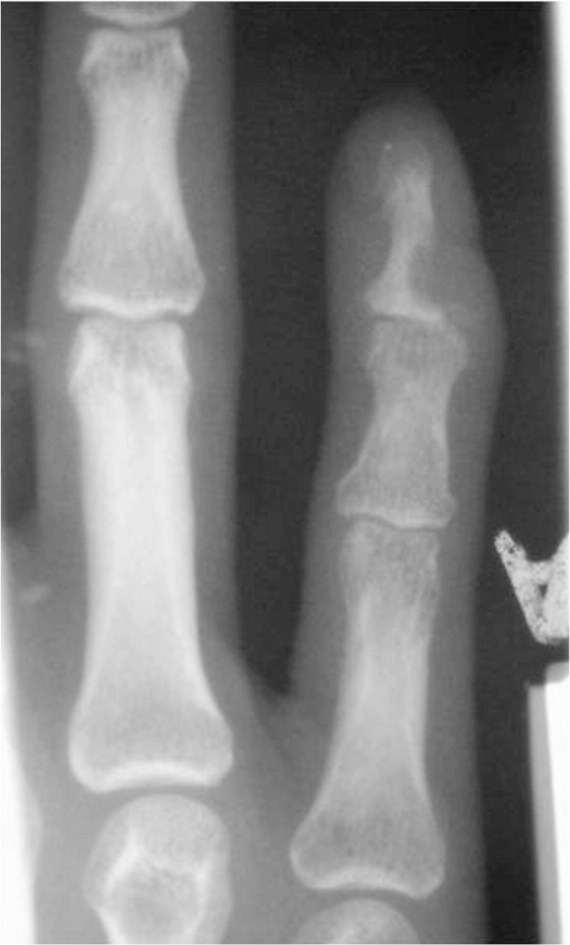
Plain radiograph with a well demarcated lytic lesion over the affected distal phalanx.
